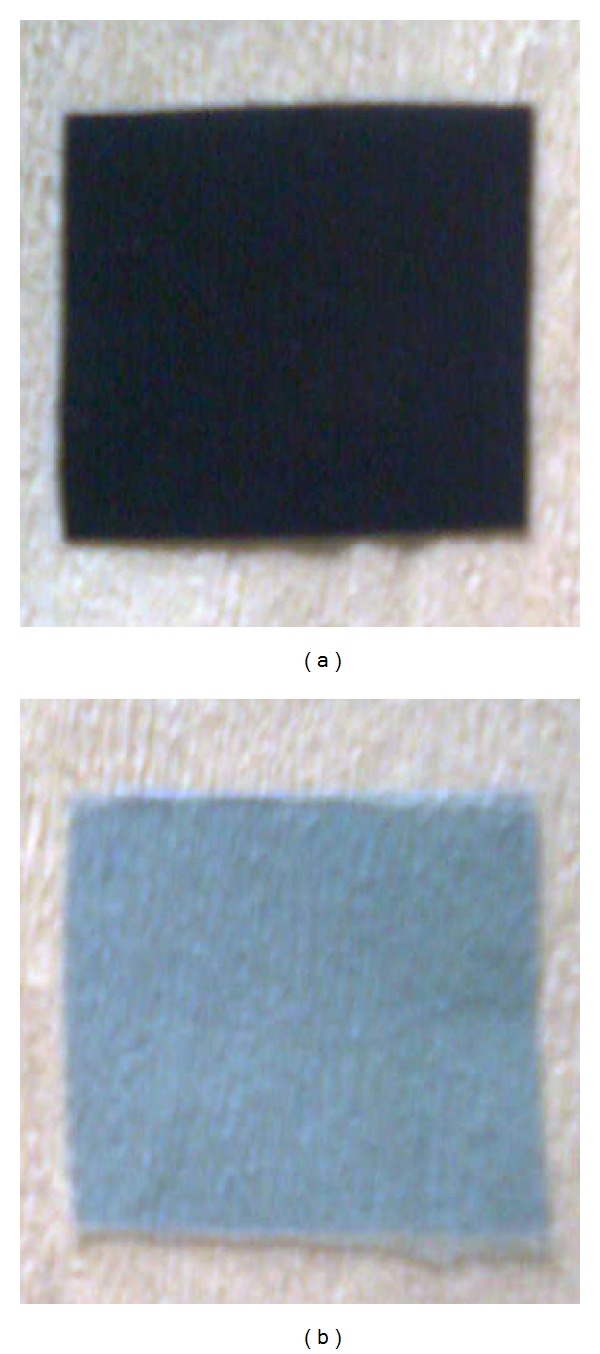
Decomposition of gelatin layer of X-ray film by protease. (a) Control; (b) experimental: X-ray film after 3 h exposure to protease.
